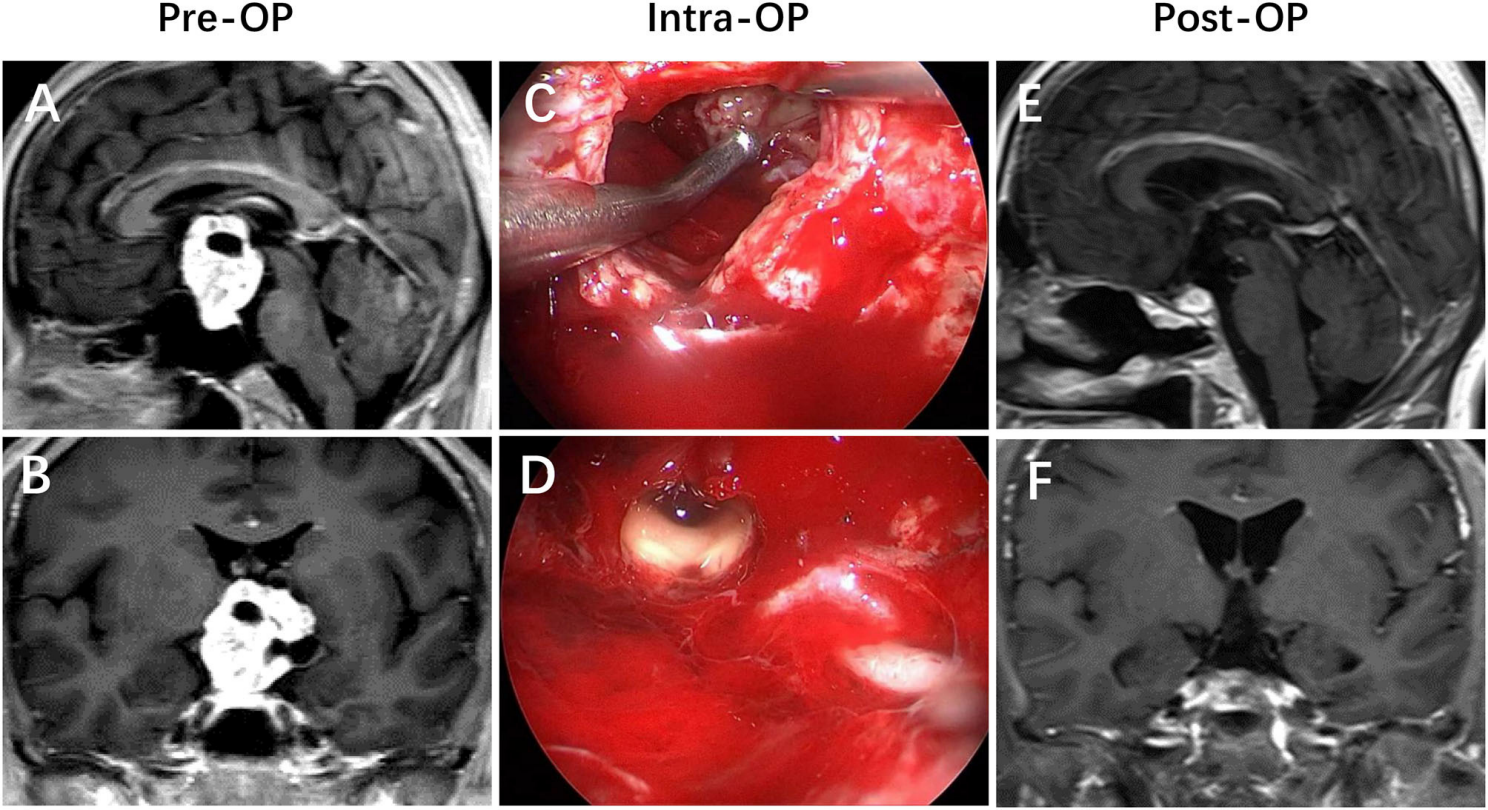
Craniopharyngioma with a large volume. (A,B) Preoperative enhanced MRI demonstrated that a giant suprasellar mass developed from the pituitary stalk that pushed the TVF upward, mimicking an intraventricular position. (C,D) Intraoperative views revealed that the patient achieved a gross-total resection of the lesion *via* the endonasal endoscopic endonasal infrachiasmatic corridor. (E,F) Postoperative enhanced MRI revealed that the lesion was totally removed, with the TVF opened.
